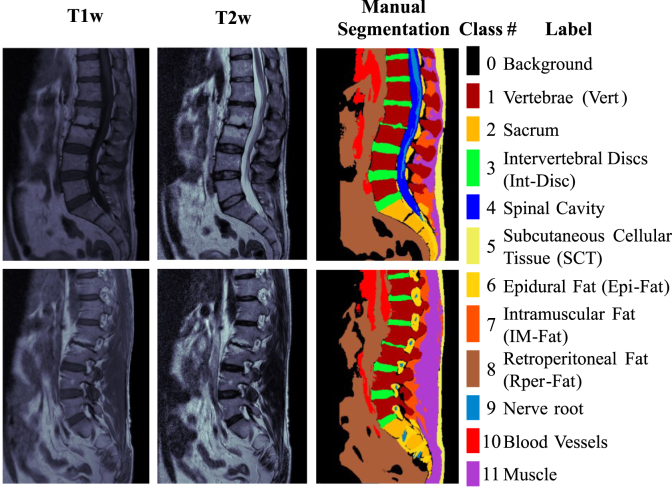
Example of two different slices with corresponding bit masks merged into a single-colored MR image using one different color per class. From left to right: T1w and T2w MR images, ground-truth semantic segmentation, and label summary. (For interpretation of the references to color in this figure legend, the reader is referred to the web version of this article.)
